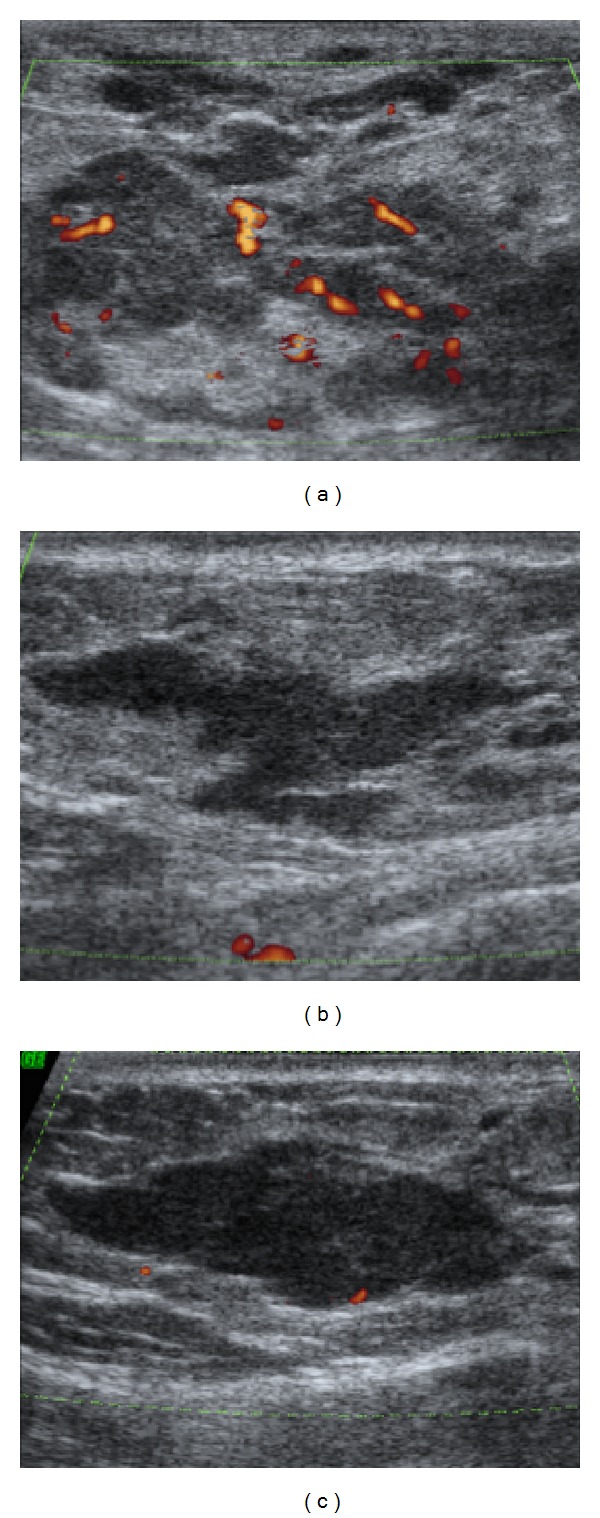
Breast ultrasonography*: ultrasonography of the left breast confirmed a hypoechoic, irregular, indistinct mass, with internal vascularity, measuring 47 × 16 mm (a). Ultrasonography of the right breast depicted two irregular, hypoechoic, circumscribed, parallel to the skin masses measuring 29 × 9 mm and 17 × 8 mm in the lower outer quadrant, near the inframammary fold (b and c).
